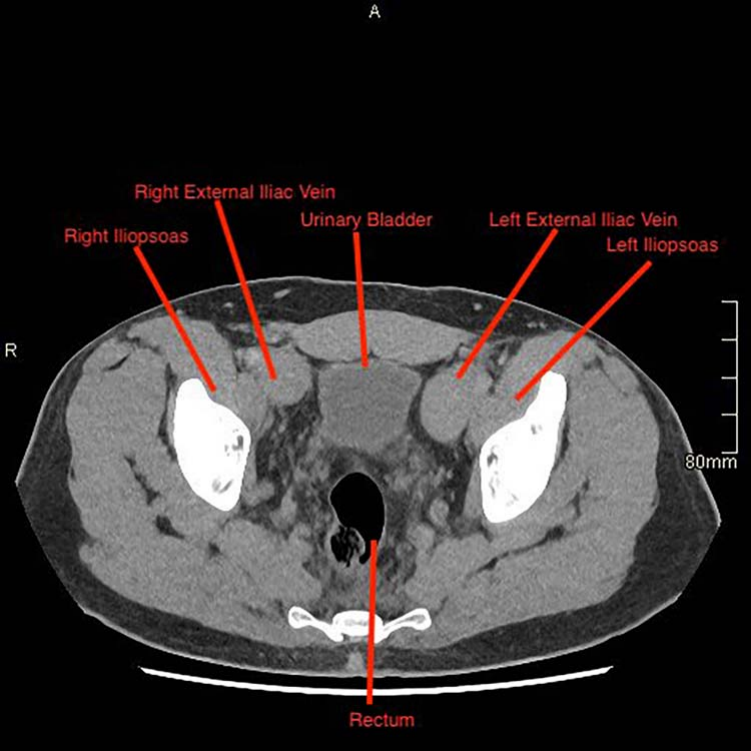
Sagittal CT image demonstrating an absent IVC.
